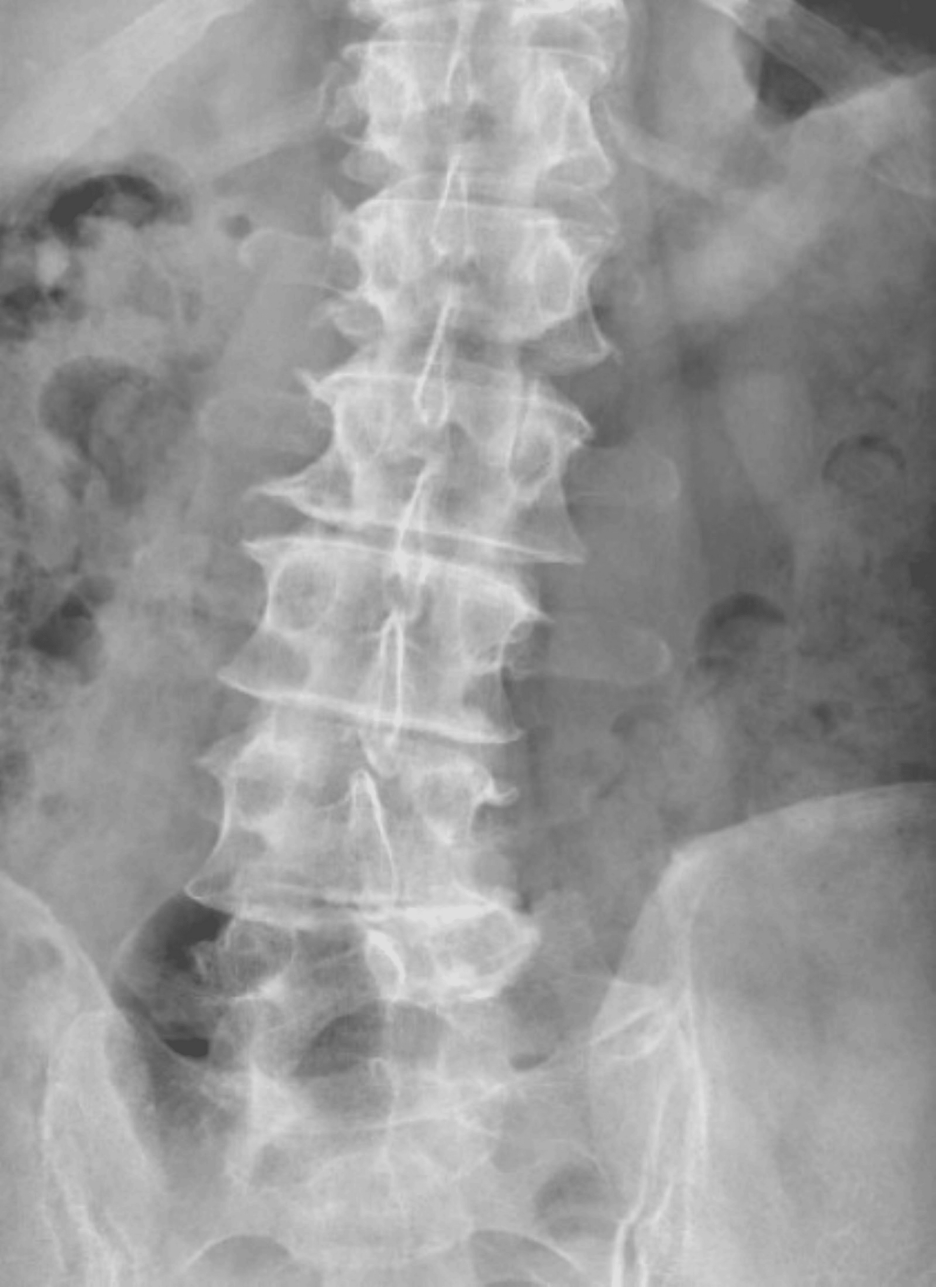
Anteroposterior lumbar X-ray demonstrating degenerative lumbar scoliosis
